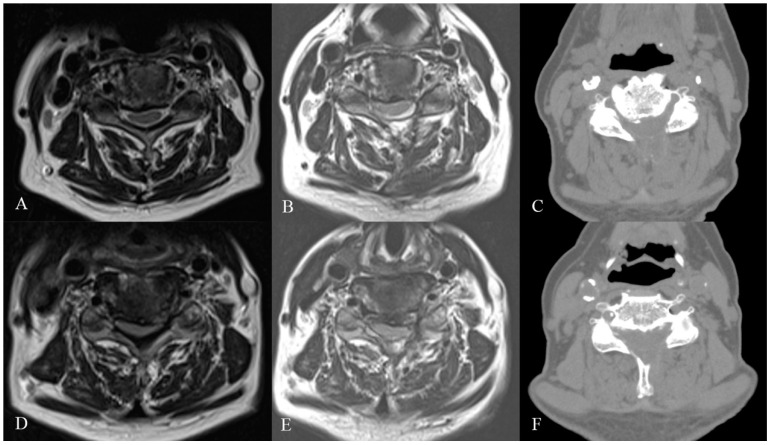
Panels (A–C) respectively represent preoperative MRI, postoperative MRI, and postoperative CT images of the C4-5 level. Panels (D–F) correspond to the preoperative MRI, postoperative MRI, and postoperative CT images of the C5-6 level.
